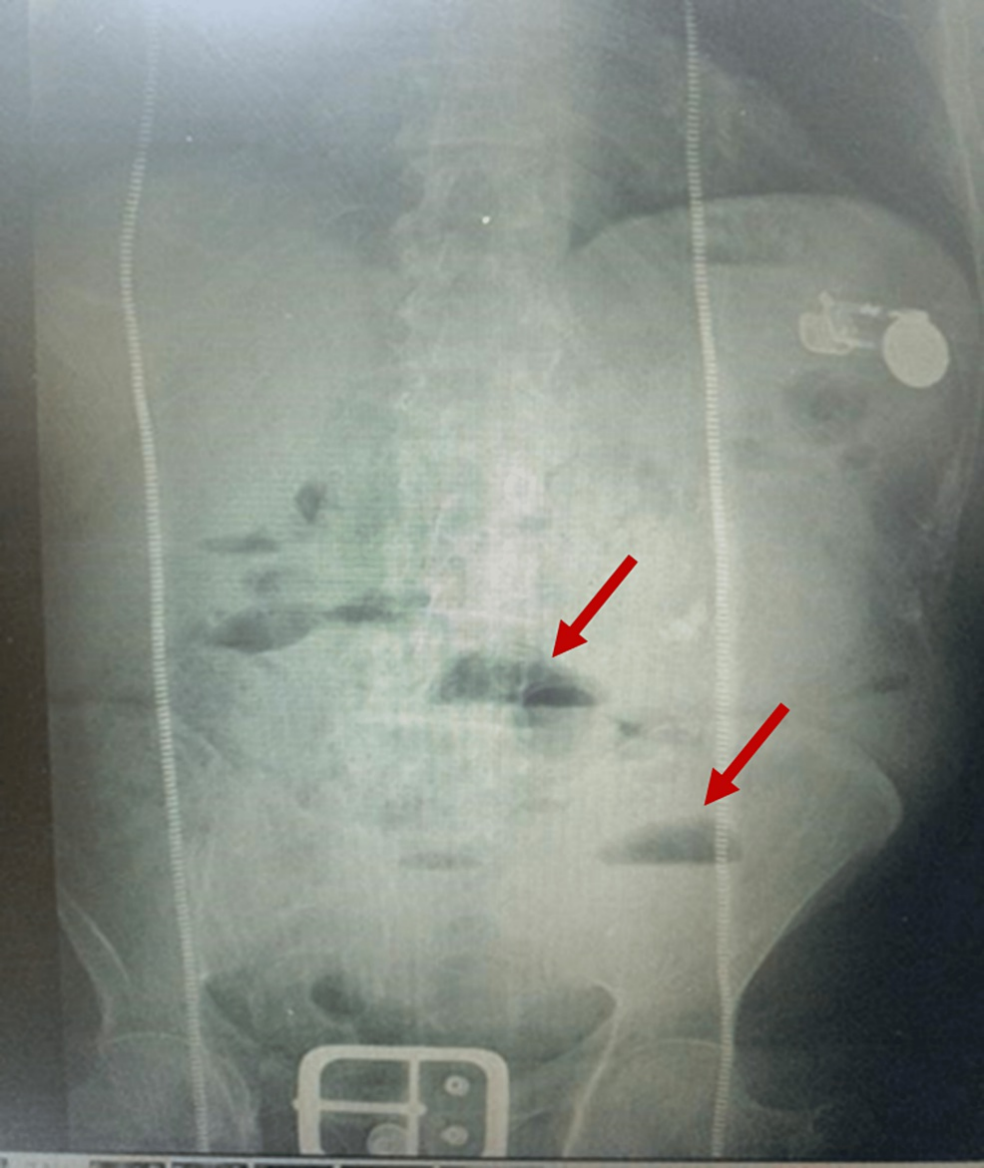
A plain abdominal X-ray revealed multiple air-fluid levels (red arrows) with dilated small bowel loops, suggestive of intestinal obstruction.
